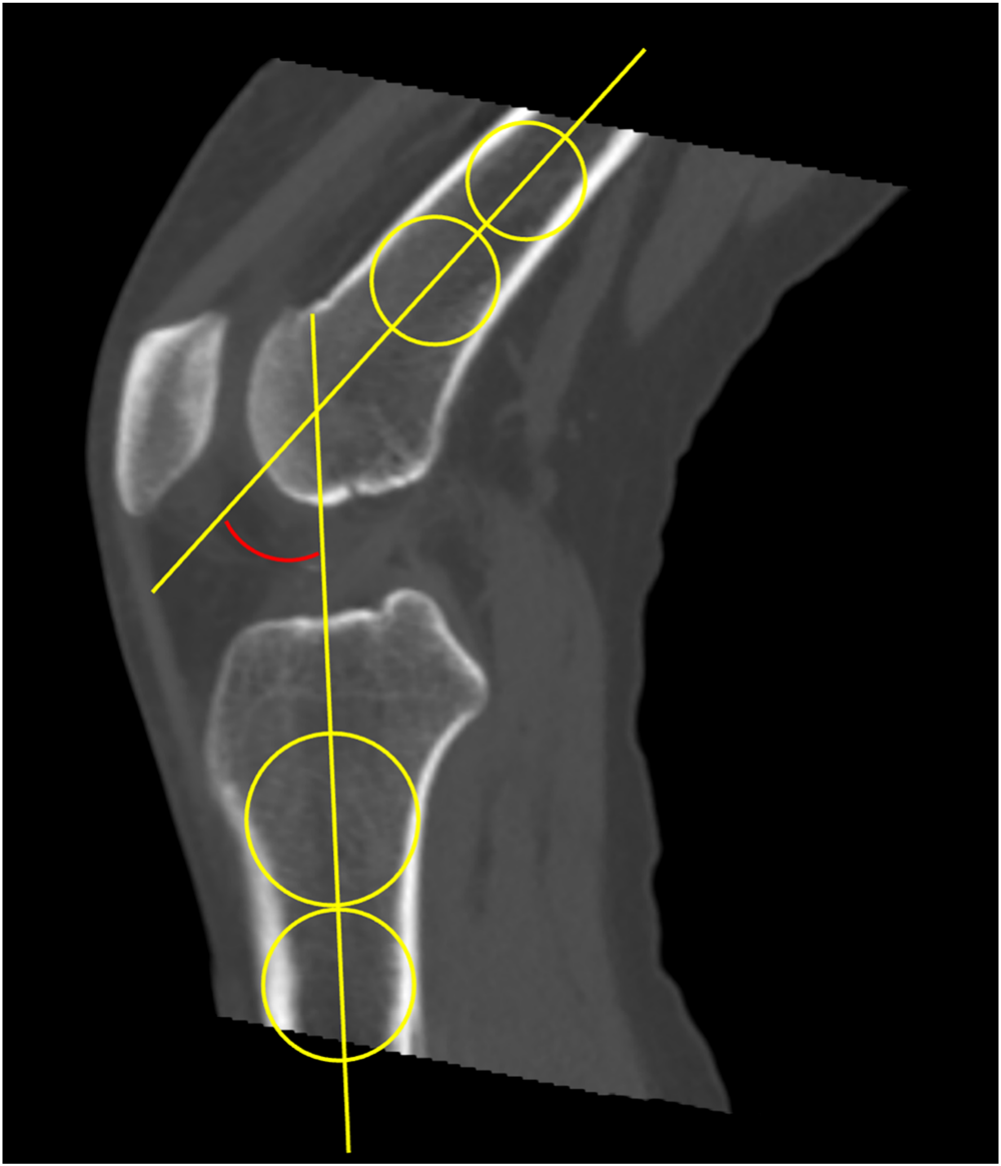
The flexion angle at each captured image was defined on sagittal dynamic kinematic computed tomography images as the angle formed by intersecting lines drawn along the centre of the femoral shaft and the tibial shaft. Hyperextension was recorded as negative value.
